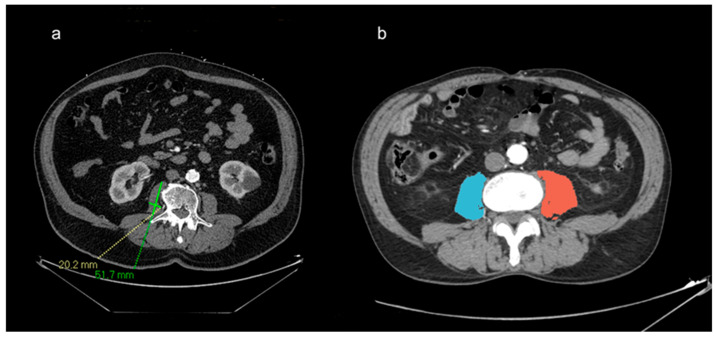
(a) Right psoas muscle diameter measured on a single axial CT slice at the level of the L3 transverse process by considering the largest diameter perpendicular to the longest anteroposterior axis of the muscle. (b) Area of both psoas muscles segmented with 3D Slicer version 5.1.0. software using muscle-specific thresholds.
